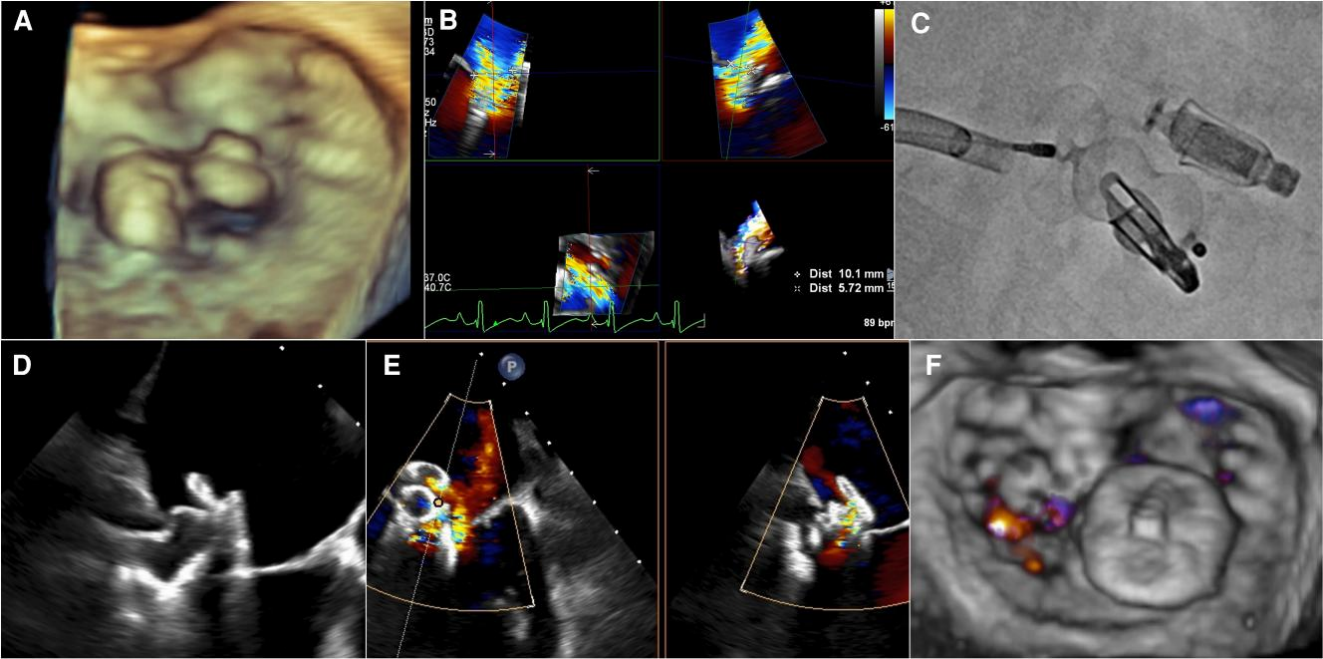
TEE and fluoroscopic images during the bail-out procedure. A hole was created by implanting the PASCAL ACE device (*A*). Based on the 3D multiplanar reconstruction image measurement (*B*), a 16-mm Amplatzer Vascular Plug II device was deployed between the devices (C–D). TEE showing only mild MR postprocedure (E–F). MR, mitral regurgitation; TEE, transoesophageal echocardiography.
